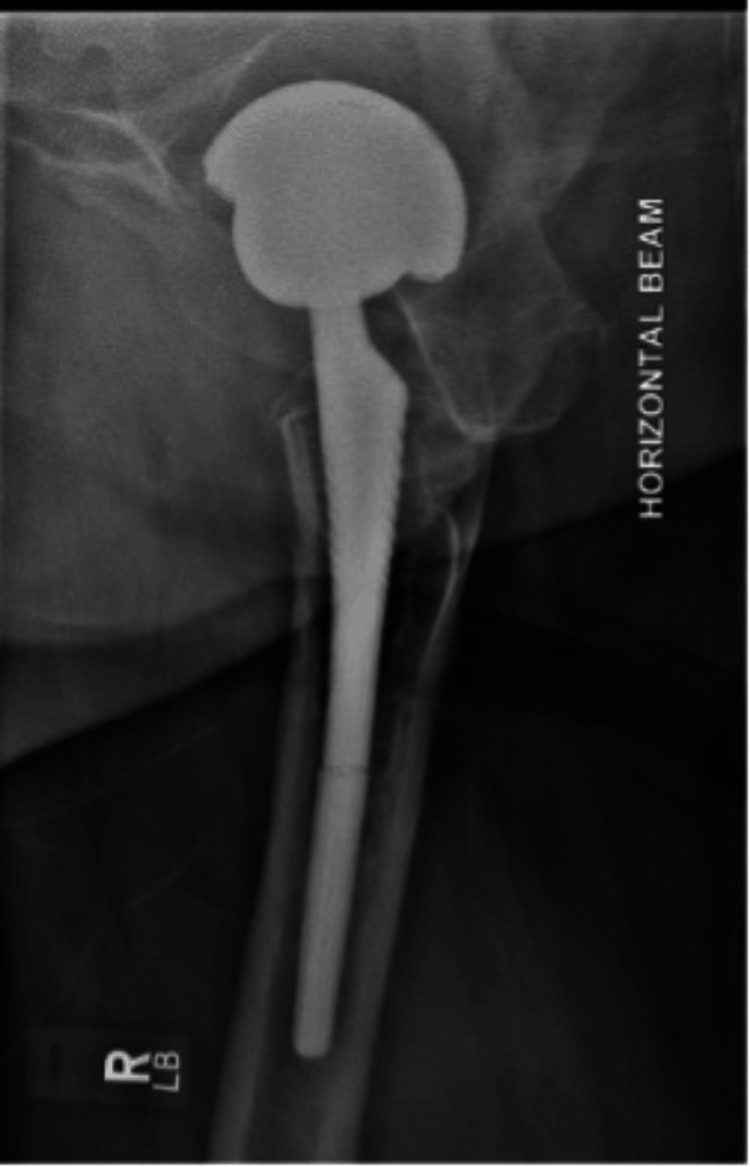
Lateral X-ray of the right hip showing the stem fracture
